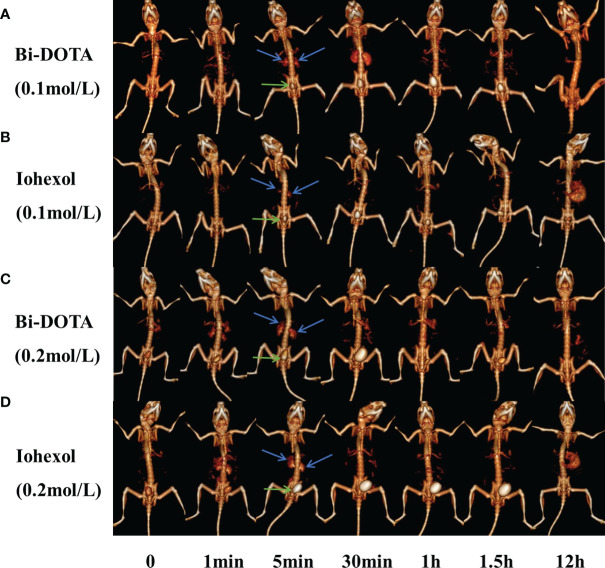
In vivo* CT urography imaging using Bi-DOTA and iohexol (blue arrows represent kidney and green arrows represent bladder). CT imaging after intravenous administration of (A) 0.1 mol/L Bi-DOTA, (B) 0.1 mol/L iohexol, (C) 0.2 mol/L Bi-DOTA, and (D) 0.2 mol/L iohexol.
